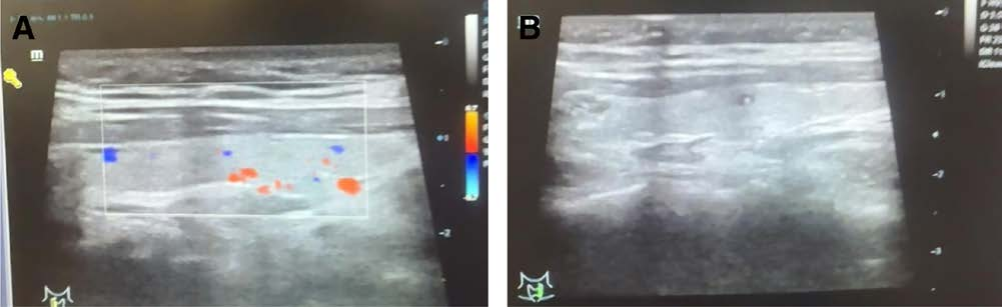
Thyroid color Doppler ultrasound. (a) Right lobe. (b) Left lobe.
